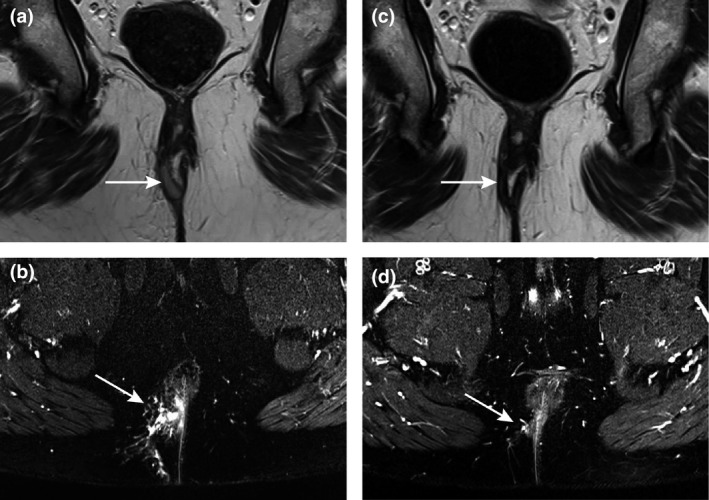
Predominantly fibrous tract at 5 months’ follow‐up. Coronal T2‐weighted images (a, c) and axial fat‐suppressed T2‐weighted images (b, d) at baseline (a, b) and at 5 months’ follow‐up (c, d) of a 37‐year‐old man. MRI showed a fistula tract that changed from predominantly granulation tissue to predominantly fibrous 5 months after the ligation of the intersphincteric fistula tract procedure, and the hyperintensity changed from pronounced to mild. Also, a focal inflammatory mass (b) was seen at baseline which disappeared at follow‐up (d). The patient showed clinical healing at the time of the follow‐up MRI.
